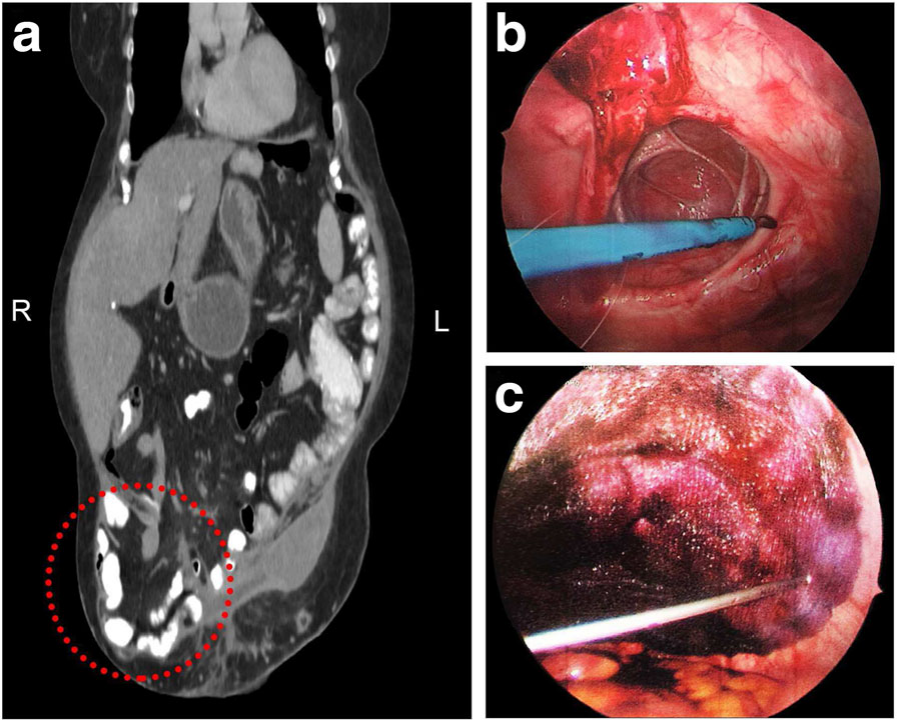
a Coronal view of the computerized tomography (CT) scan. Laparoscopic view of a 4-cm ventral hernia before b and after c closure with Ventrio ST mesh. Red ellipse = hernia; R = right; L = left
